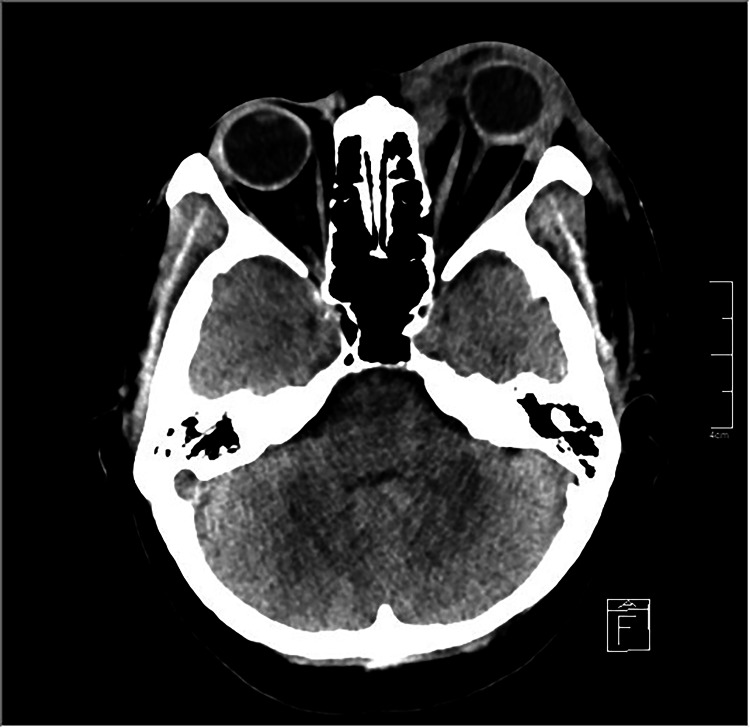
Plain CT image of the orbit, axial view The left eye appears proptosed with the presence of an enhancing collection in the left orbit located medially in the extraconal space. The preseptal soft tissue is thickened with the presence of fat streakiness. The globe and its content are preserved. The ethmoidal sinus is clear, and no enhancing focal brain parenchymal lesion is seen.
